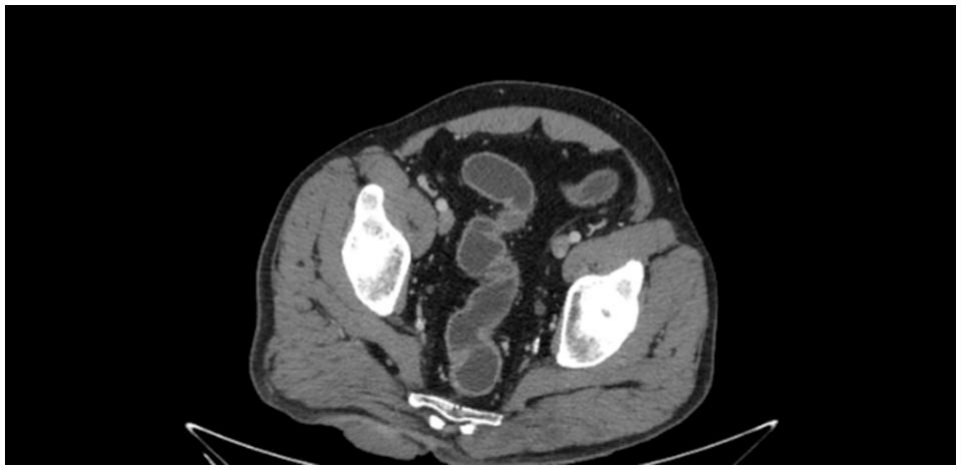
The current patient has underwent an axial CT showing subcutaneous gluteal mass with overlying skin thickening and no muscular invasion. This image was captured during a CT scan the patient has underwent in the Institute.
